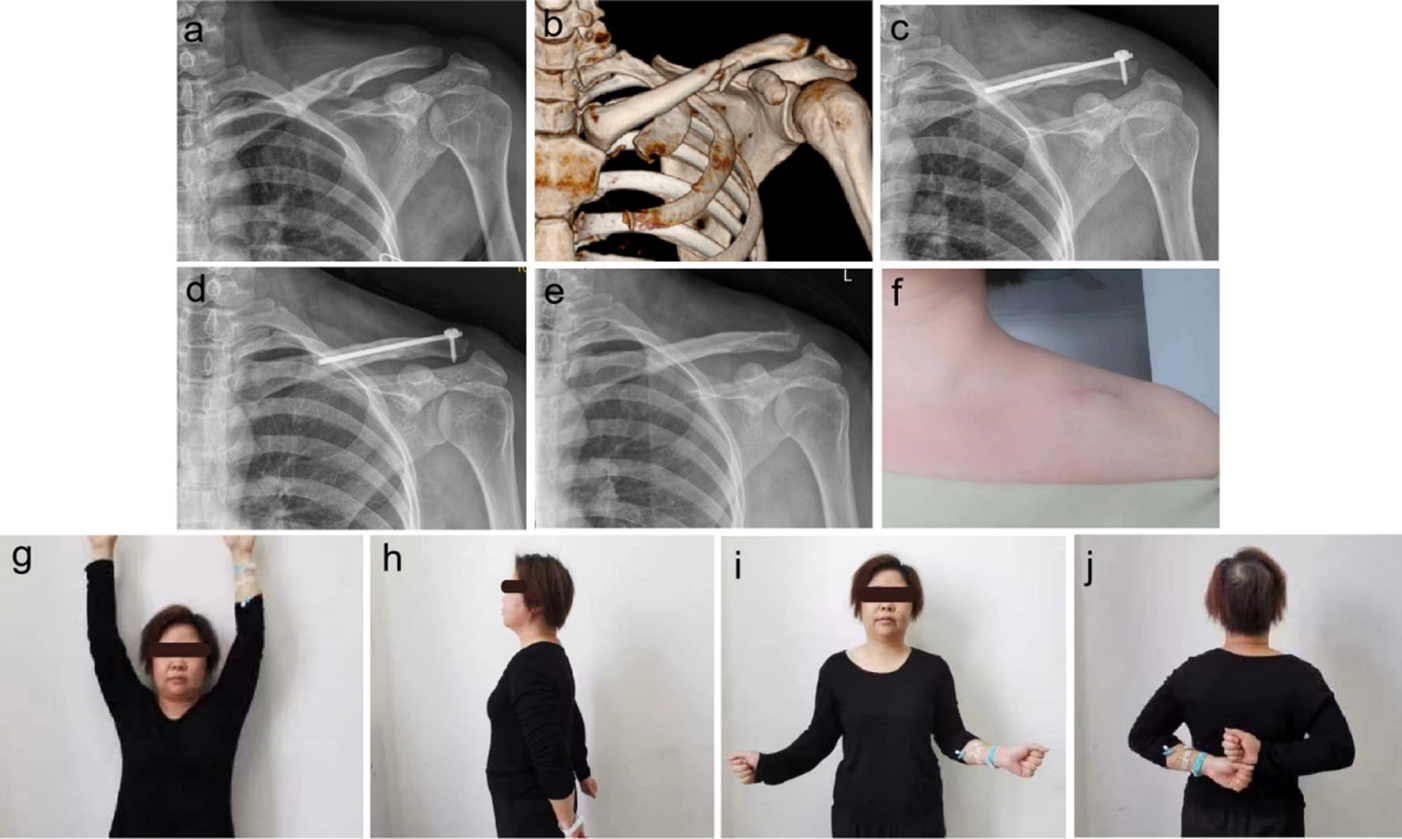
Case of a 34-year-old female who experienced a traffic accident. a, b The preoperative orthoptic radiograph and CT 3D reconstruction of the left clavicle showed a mid-clavicle fracture. c At 2 d postoperatively, an orthoptic radiograph of the left clavicle showed a well-replaced fracture. d At 10 d postoperatively, the skin at the incision was esthetically pleasing, with no scars. e At 7 months postoperatively, an orthoptic radiograph of the left clavicle showed good fracture healing. f At 7 months postoperatively, an orthoptic radiograph of the left clavicle after removal of the internal fixation showed a normal clavicle morphology. g–j By 7 months postoperatively, the function of the shoulder joint had returned to normal
